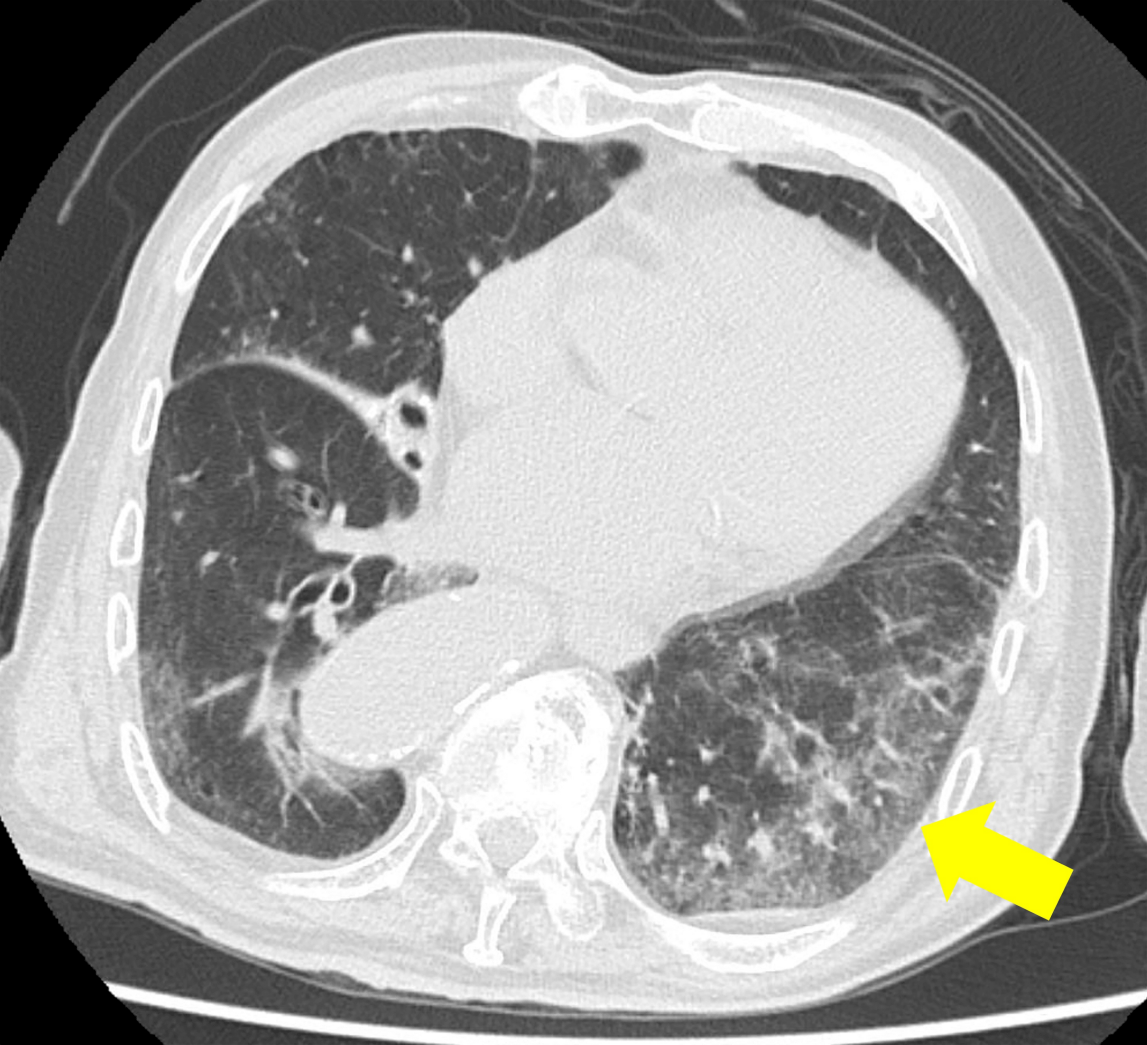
Computed tomography (CT) of the chest. CT showing ground-glass opacity in the left lower lobe (yellow arrow). CT findings were not directly related to the subsequent development or diagnosis of drug-induced immune thrombocytopenia (DITP).
